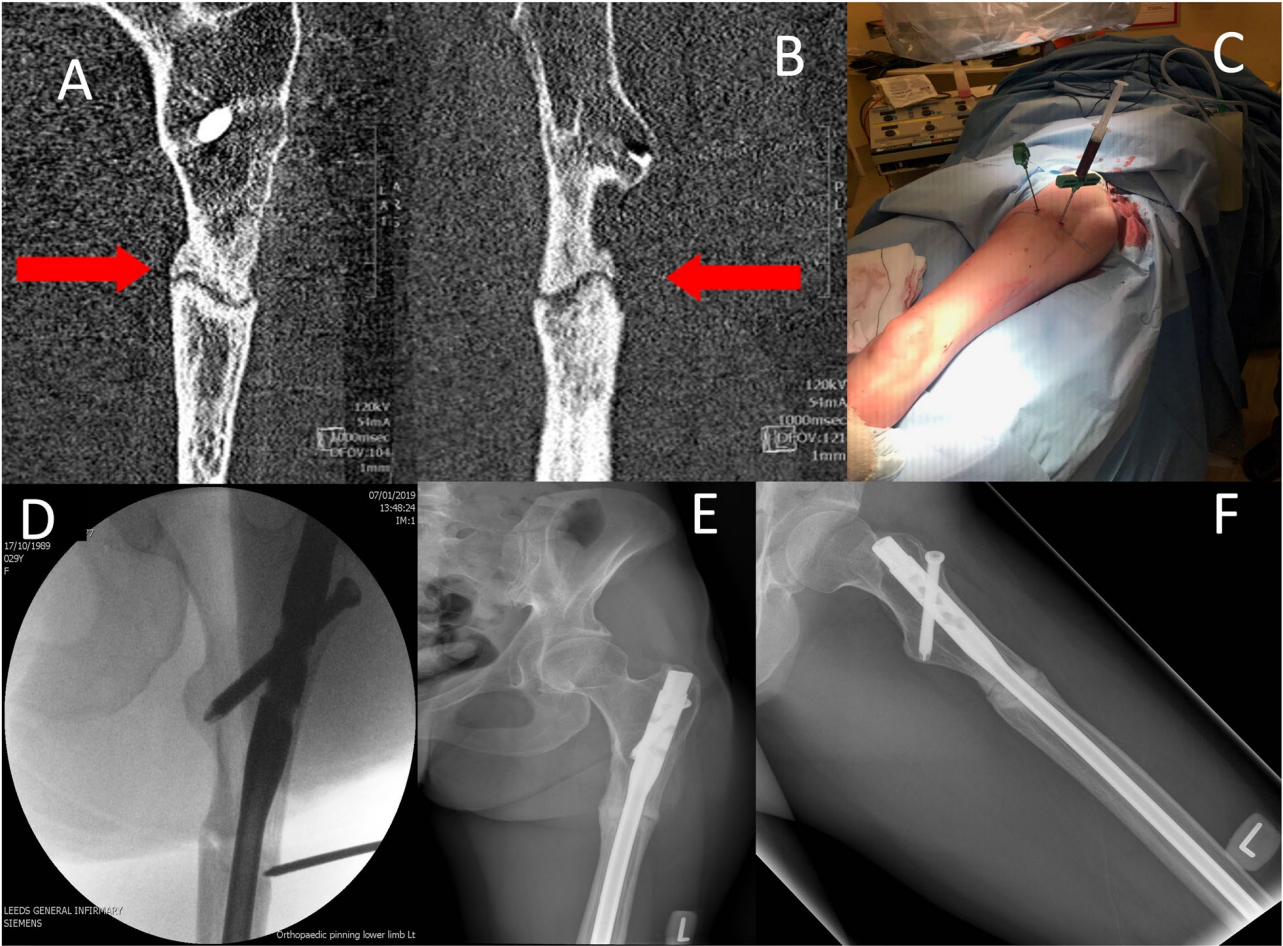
A 42-year-old male sustained a closed distal tibial fracture following a fall (A, B). The fracture was stabilised with a MIPO plate and demonstrated little callus formation 6 weeks after surgery (C, D). A CT scan taken at 6 months demonstrates an established nonunion (E). The patient underwent the harvesting of 60 ml bone marrow from the ipsilateral iliac crest, which was concentrated down to 7 ml of BMAC. This was injected by a percutaneous technique into the nonunion site (F, G). Following this technique, union was achieved within 4 months (H, I). *MIPO* Minimal invasive plate osteosynthesis
